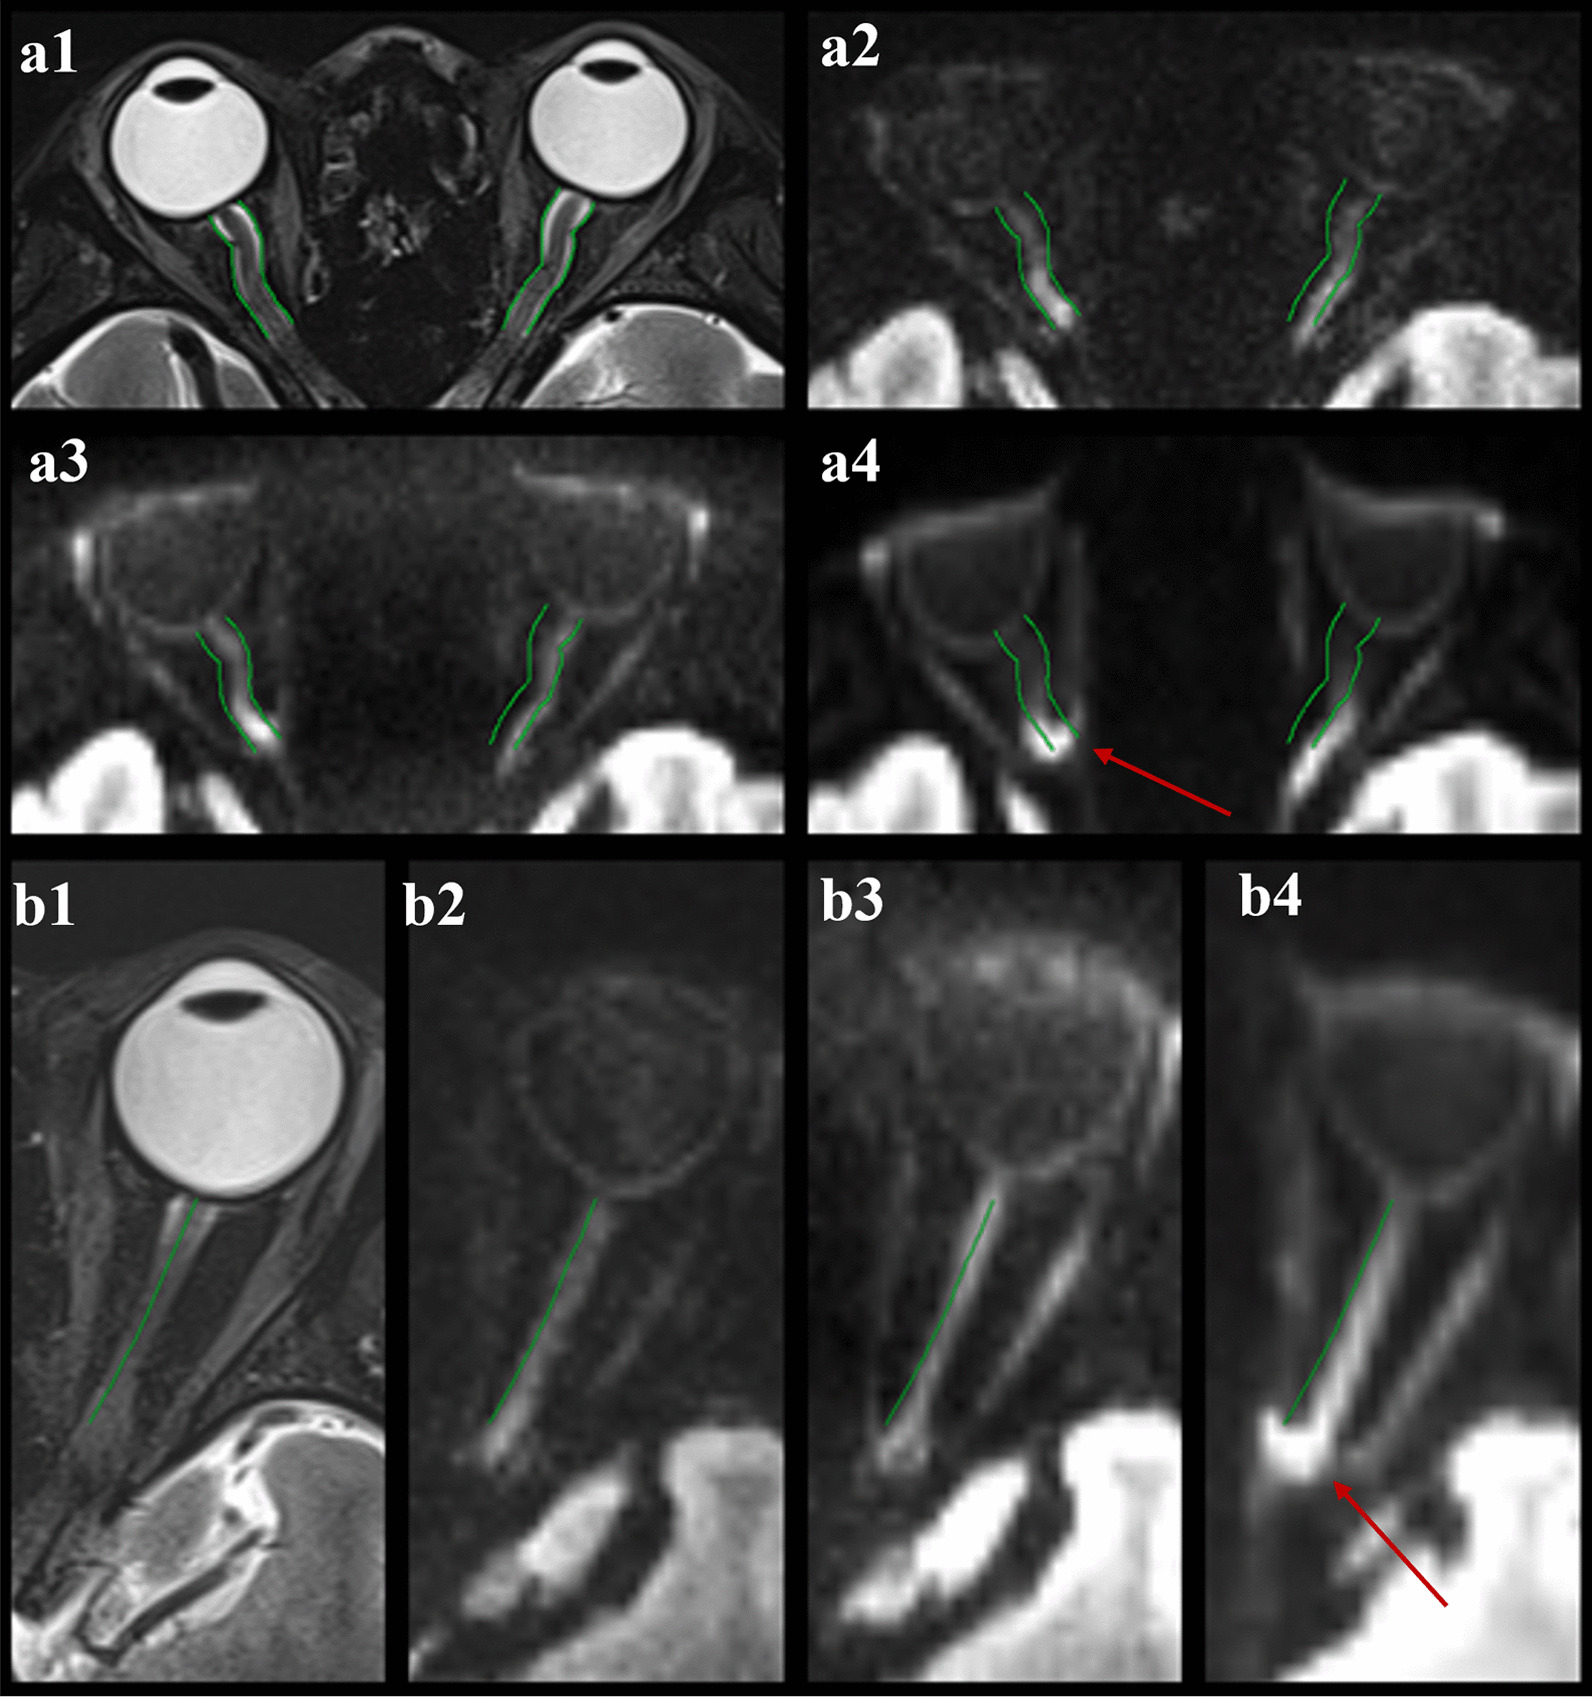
Examples of image quality and distortion of three DW techniques. a Imaging in a 28-year-old female volunteer. a1–a4 images of T2w, rs-EPI DWI at b value = 1000 s/mm^2^, rFOV-EPI DWI at b value = 1000 s/mm^2^ and ss-EPI DWI at b value = 1000 s/mm^2^, respectively. b Imaging in a 25-year-old female volunteer. b1–b4 images of T2w, rs-EPI DWI at b value = 1000 s/mm^2^, rFOV-EPI DWI at b value = 1000 s/mm^2^ and ss-EPI DWI at b value = 1000 s/mm^2^, respectively. Note the improved image quality and markedly reduced susceptibility distortions in the rs-EPI and rFOV-EPI sequences compared with ss-EPI. There are severe distortions due to the susceptibility artifacts caused by the air-bone-tissue interface on ss-EPI DWI (arrows in a4, b4). rs-EPI DWI, readout-segmented echo-planar imaging diffusion-weighted imaging; rFOV, reduced field-of-view; ss-EPI, single-shot echo-planar imaging
